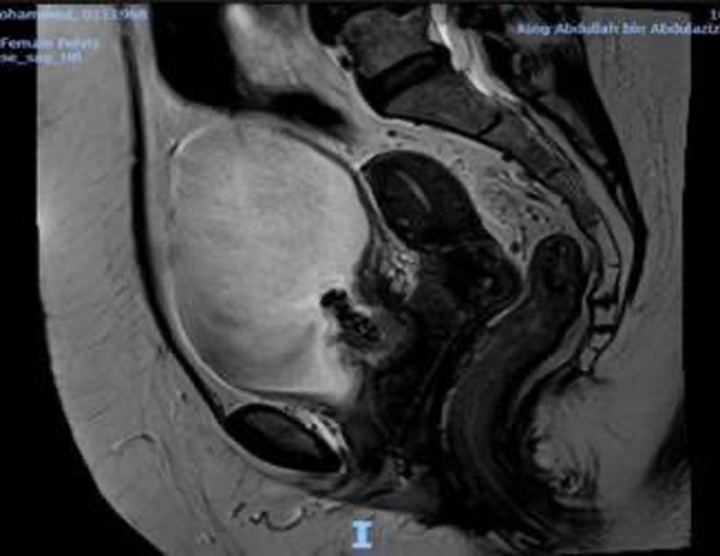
An enhanced pelvic MRI showed a defect in the base of the urinary bladder in the midline measuring ~1.2×2 cm containing heterogeneous material likely representing mesh.
